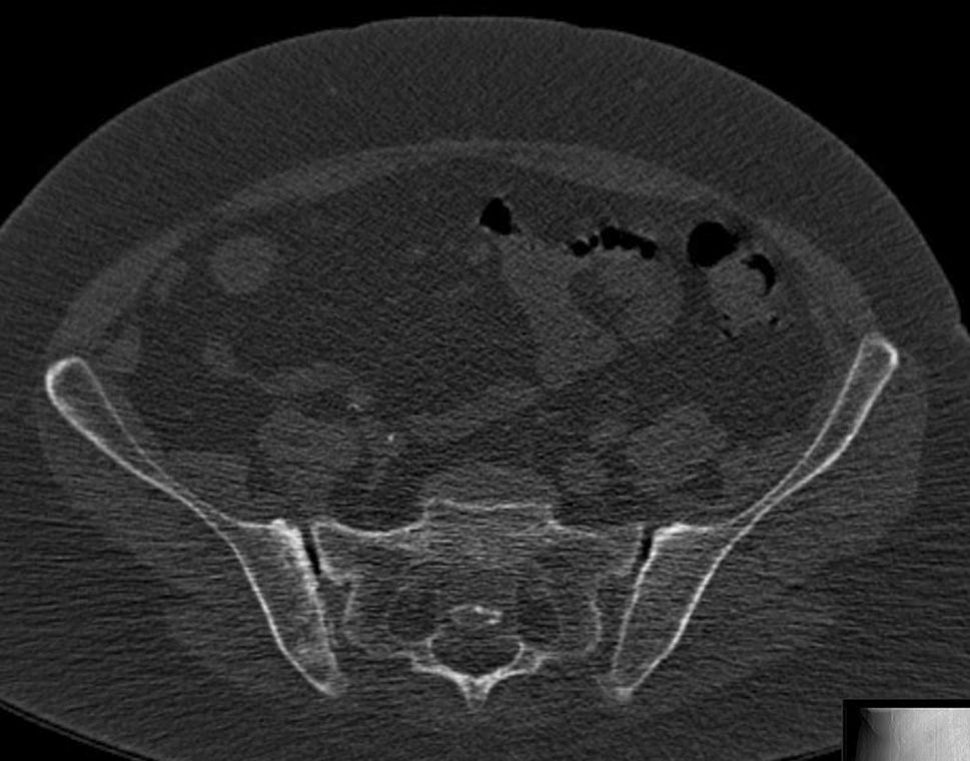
CT-slice through the posterior pelvis. CT evaluation allows for adequate visualization of the SI joint and sacrum. In this image widening of both SI joints can be seen with bilateral signs of degenerative SI joint arthrosis. The typical cortical densening can be best seen on the iliac side of the SI joint
